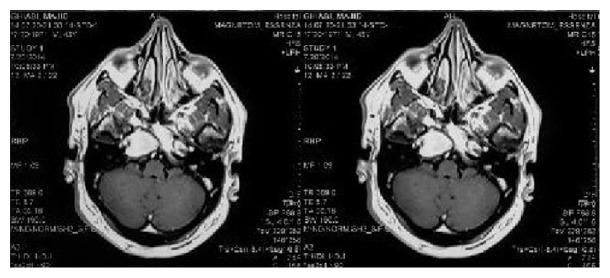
Axial post-GAD MRI showing the cholesterol granuloma involving the left petrous apex, adjacent to carotid artery.
